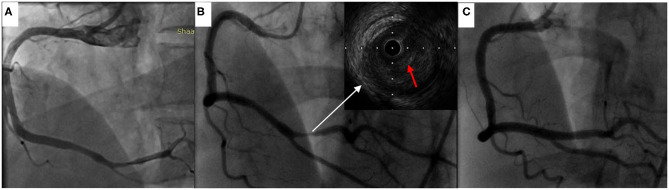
Coronary angiography and intravascular ultrasound findings. (A) The right coronary angiography results 2 months earlier. (B) Coronary angiography revealed severe stenosis in the distal right coronary artery, and intravascular ultrasound demonstrated plaque rupture and thrombosis (red arrow indicated thrombosis). (C) Coronary angiography after stent implantation showed good results.
